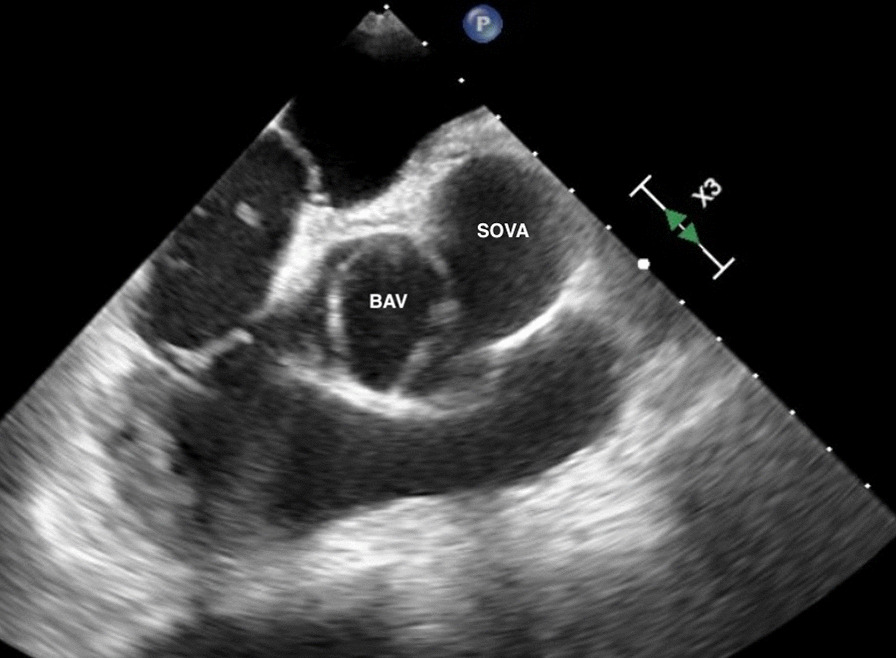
Transesophageal echocardiogram showing the bicuspid aortic valve (BAV) and the left sinus of Valsalva aneurysm(SOVA) adjacent to it
